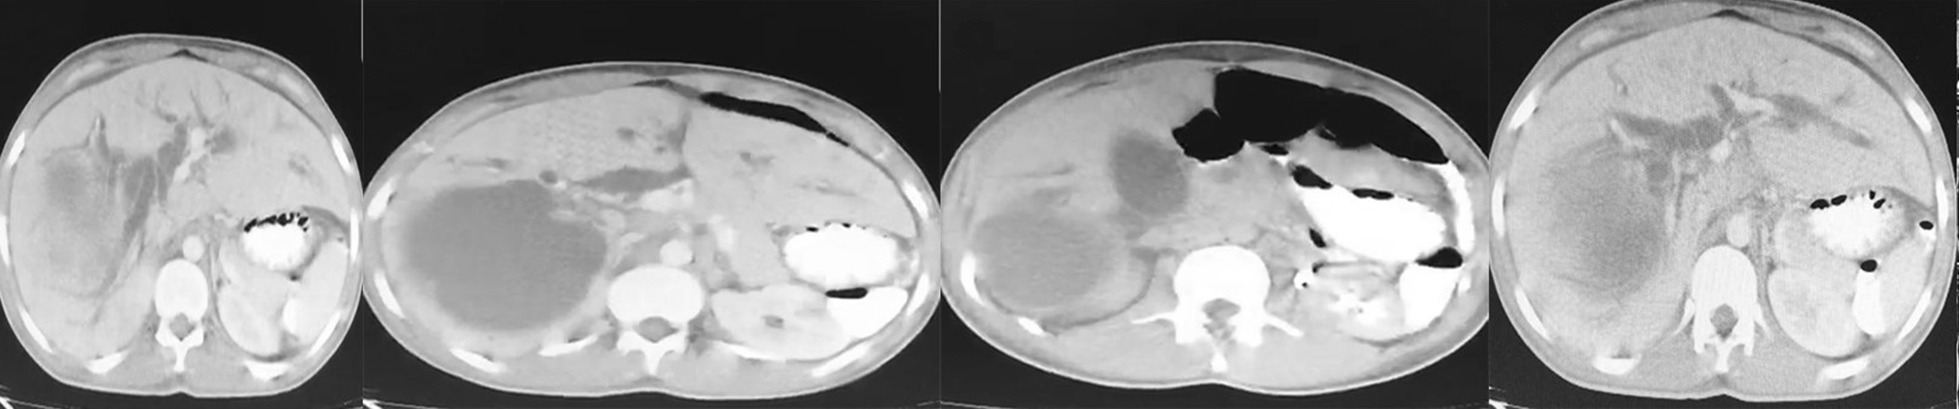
Axial contrast enhanced computed tomography scan showing unilocular hepatic cysts with detached laminated membranes compatible with hepatic hydatid cysts on segments II and IV. Intrahepatic biliary duct dilation is also seen
